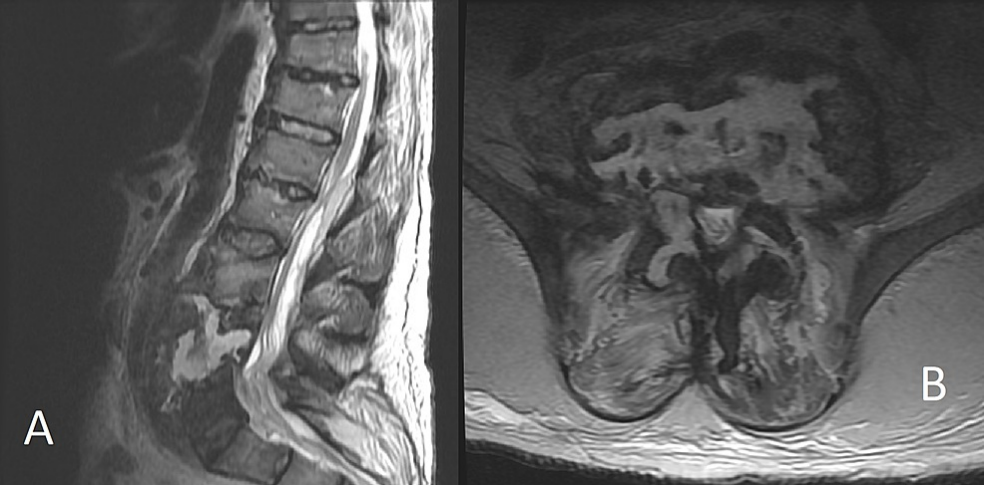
T2 sagittal (A) and axial (B) MRI of the L spine demonstrating osteomyelitis with near-complete destruction of the lumbar 4 vertebral body
